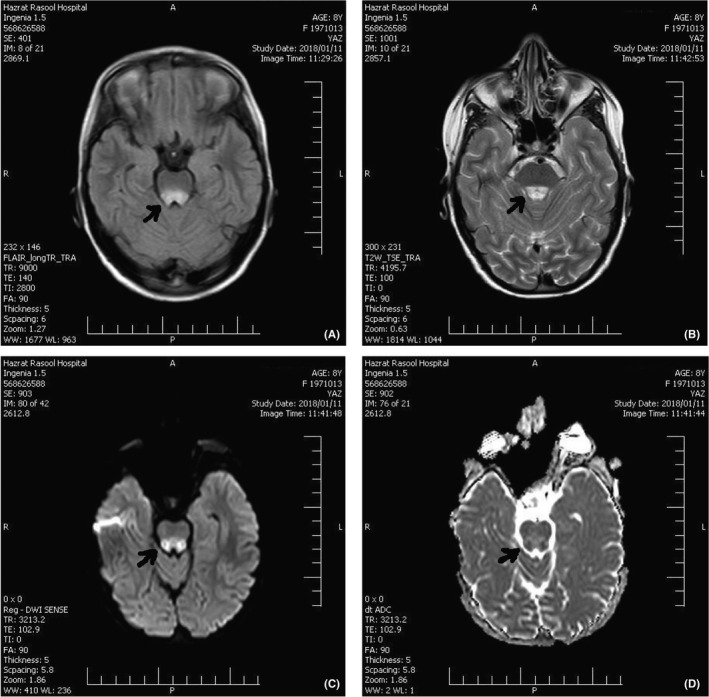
The first brain MRI. (A) Axial brain MRI (flair): Arrow shows hyperintensity in dorsal midbrain; (B) Axial brain MRI (T2): Arrow shows hyperintensity in dorsal midbrain; (C) Axial brain MRI (DWI): Arrow shows hyperintensity in dorsal midbrain; (D) Axial brain MRI (ADC map): Arrow shows restriction in dorsal midbrain involvement
